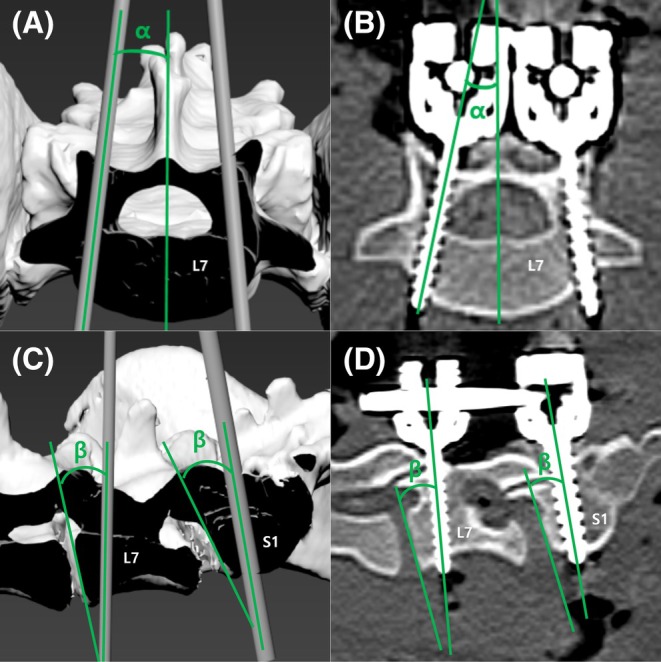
Assessment of transverse and sagittal angular deviation for pedicle screw placement. Transverse plane images of the L7 vertebra showing the planned transverse angle (α) on (A) the preoperative three‐dimensional (3D) model and (B) the actual angle on the postoperative computed tomography (CT) image. Angle α was defined as the angle between the sagittal plane and a line along the screw axis. Sagittal plane views showing the planned sagittal angle (β) on (C) the preoperative 3D models of L7 and S1 and (D) the actual screw positions on postoperative sagittal CT reconstructions. Angle β was defined as the angle between a line along the cranial vertebral endplate and the screw axis in the sagittal plane. Angular deviation was determined by comparing preoperative plans with postoperative screw.
